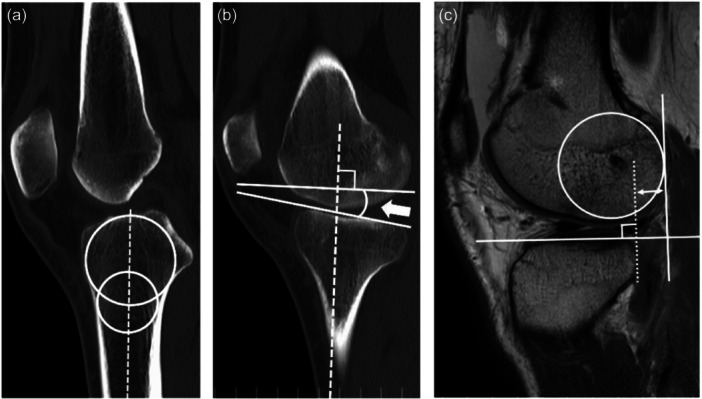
Measurement of posterior tibial slope and anterior translation of tibia (magnetic resonance imaging, computed tomography). (a, b) Measurement of posterior tibial slope (PTS). Two circles were drawn, which best fit in the proximal tibia. The centre of the caudal circle was positioned on the circumference of the cranial circle. Dotted line shows the tibial axis, which connects the centre of two circles and the arrow shows the PTS relative to the tibial axis. (c) Measurement of anterior tibial translation in magnetic resonance imaging and computed tomography. The line was drawn along the margin of the posterior margin of the tibia, which is perpendicular to the tibial plateau (dotted line). A best‐fit circle was drawn over the subchondral line of the posterior condyle, and a line perpendicular to the tibial plateau was then drawn along the posterior margin of the circle. The distance between the two lines was measured.
